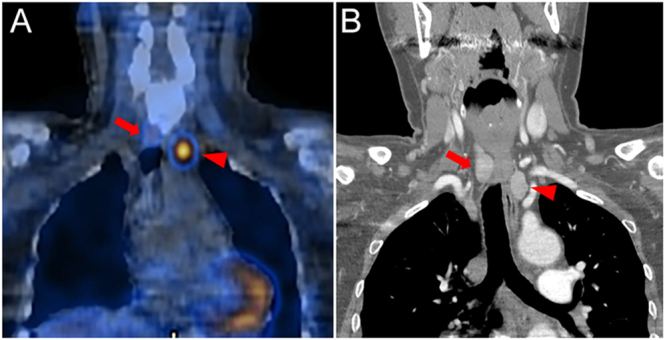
Cervical ultrasonography demonstrated a hypoechoic lesion in the middle dorsal of the right thyroid lobe (A, white arrowhead), measuring 2.5 × 1.6 cm, which was suspicious for parathyroid carcinoma (PC) because of its irregular morphology. There was another hypoechoic image below the left thyroid lobe (B, white arrowhead). It measured 2.0 × 1.3 cm, with a clear border and regular shape, which was considered a parathyroid adenoma (PA).
